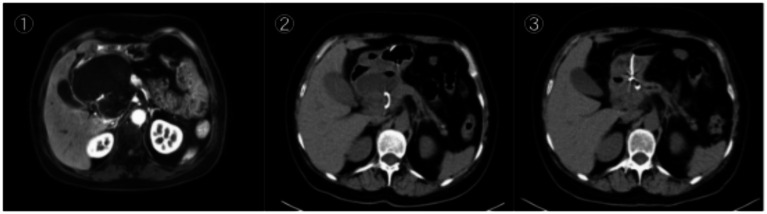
(①) Preoperative abdominal enhanced CT suggested a cystic lesion with a diameter of about 8.5 cm in the body and tail of the pancreas; (②) abdominal CT performed 2 weeks postoperatively showed a significant reduction in the pancreatic cyst (diameter 3.2 cm); (③) abdominal CT performed 4 weeks postoperatively showed a further reduction in the pancreatic cyst (diameter 1.5 cm) with almost complete disappearance of cyst fluid.
